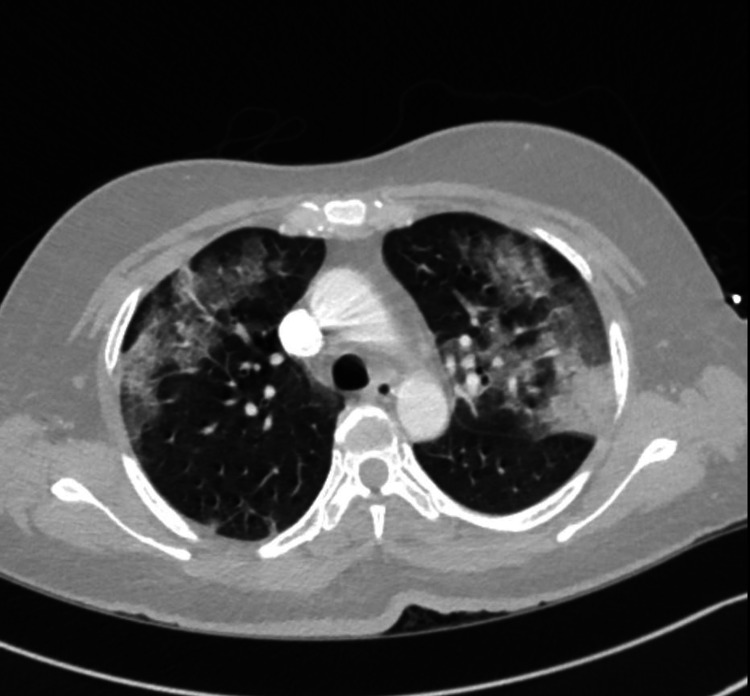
CT angiography showed diffuse bilateral ground glass opacities consistent with COVID 19 pneumonia
